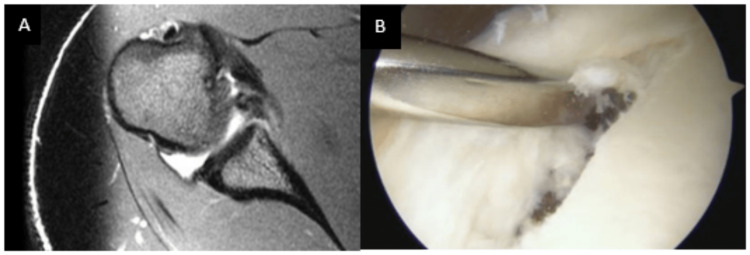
Preoperative and intraoperative images of anterior labral tears A. Axial T2 MRI of a right shoulder demonstrating a displaced anterior labral tear. B. Right shoulder displaced anterior labral tear in the lateral decubitus position when viewing from the anterior superior arthroscopic portal.
